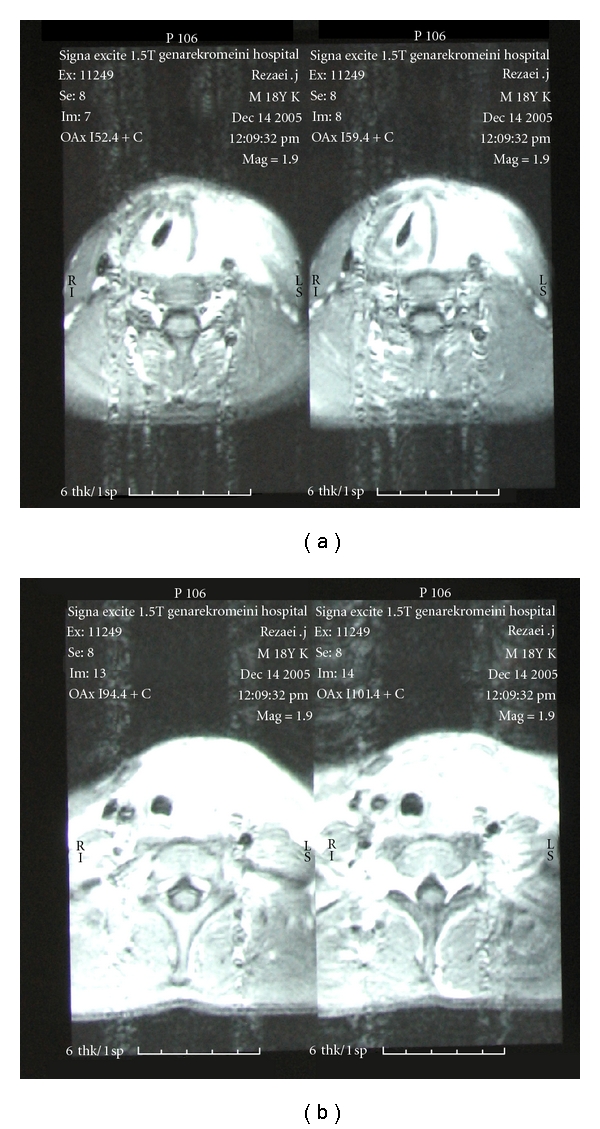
MRI findings in the second case.
